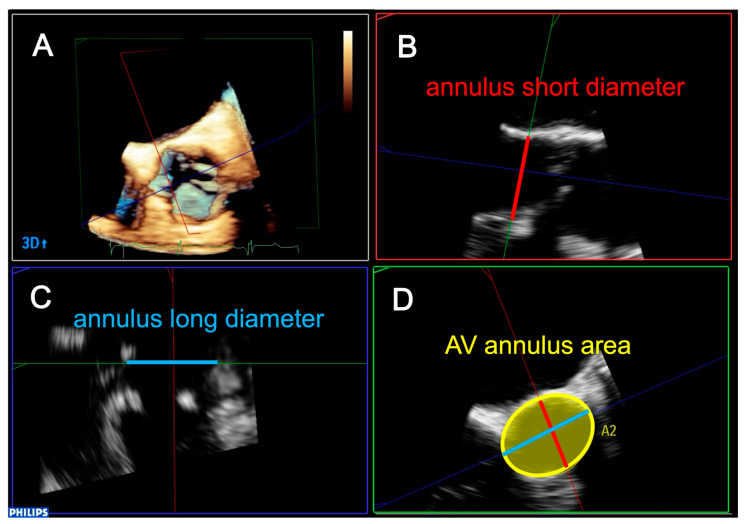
Methods of three-dimensional analysis of aortic valve using transesophageal echocardiography. From preoperative three-dimensional transesophageal echocardiographic (3D TEE) data, aortic annulus area was measured using 3DQ software at mid-diastolic phase (A). Two-dimensional (2D) long-axis view: aortic annulus short diameter (red line) (B) and long diameter view (blue line) (C). 2D short-axis view (D), AV annulus area (yellow circle). AV; aortic valve.
